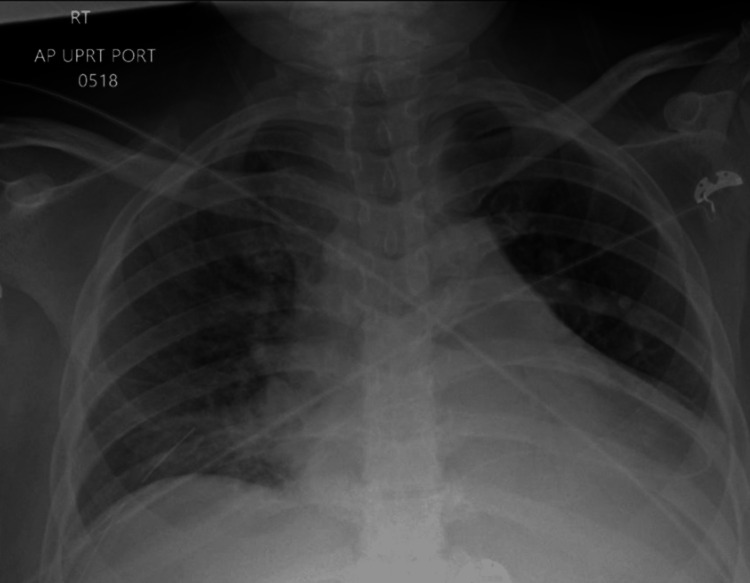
Chest X-ray showing fluid overload (worse on the left side).
